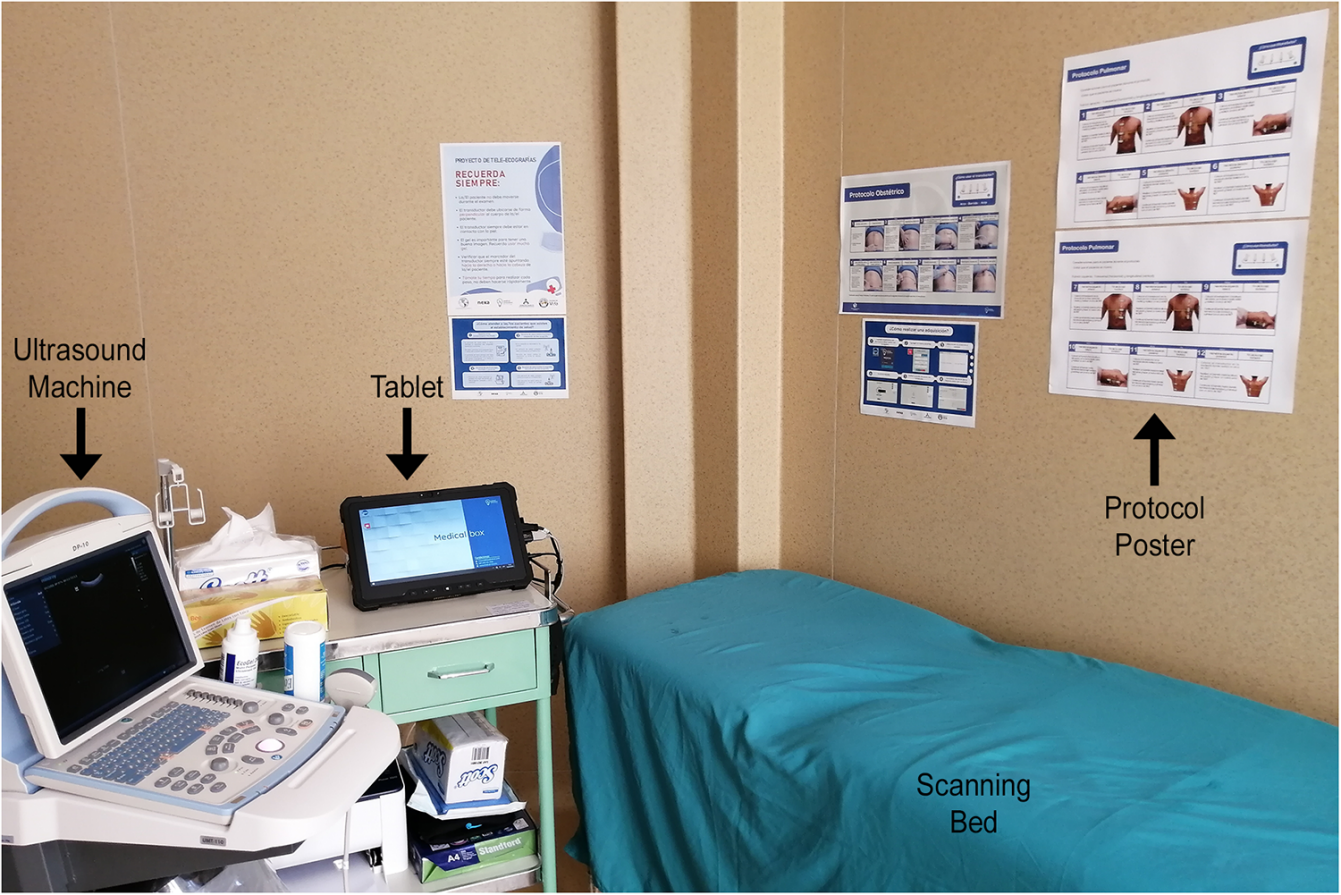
Example teleultrasound station. Labeled photo from the teleultrasound station at Ticlacayán demonstrating the required setup. The tablet connects to the ultrasound machine and guides the user to input the clinical history and perform the VSI protocol. Posters on the wall remind the operator how to perform the protocol.
